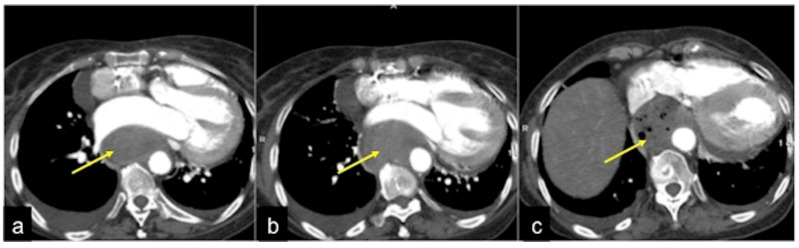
CTA images of Mallory–Wess lesion in the arterial phase. Oedematous thickening oesophageal wall ((a,b) arrow) with small gas bubbles around the laceration site (c, arrow).
